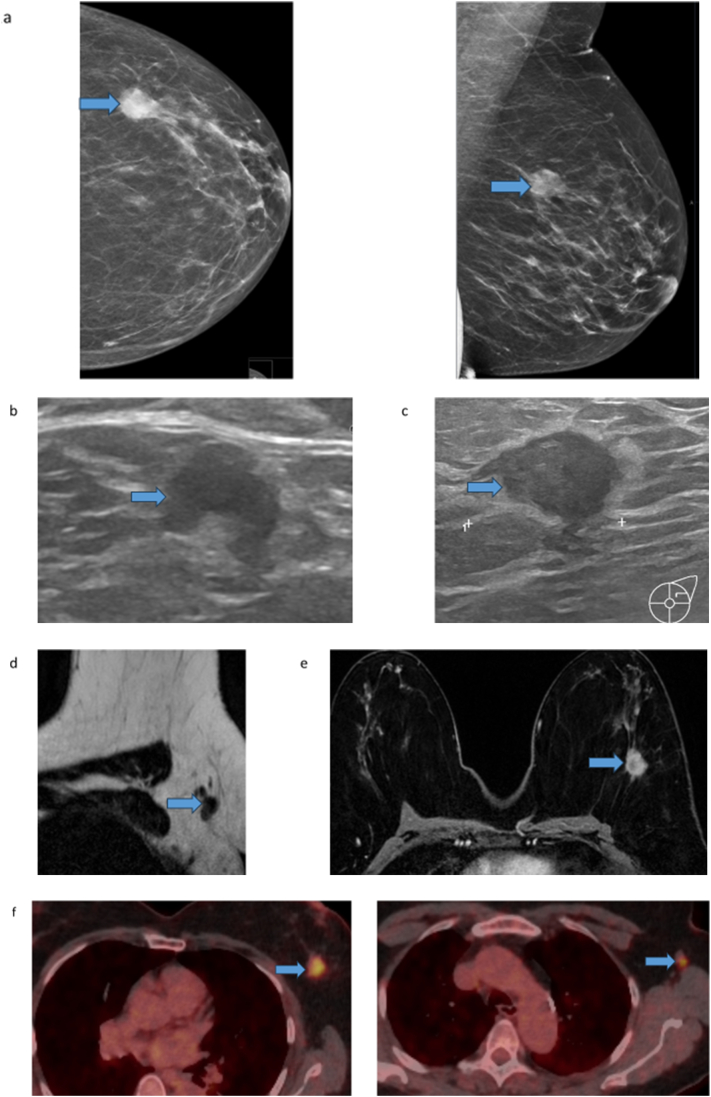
Mammography of the left breast shows a spiculated mass in the upper lateral quadrant in front and oblique view, suspicious of malignancy (blue arrow) (a). Ultrasound of the left axilla demonstrates a pathological axillary lymph node with a cortical thickness of 5 mm (blue arrow), which is highly suspicious for metastasis (b). Ultrasound of the left breast reveals a malignant irregular hypoechoic mass in the left breast (blue arrow) (c). MRI of the left axilla demonstrates a pathological lymph node with cortical thickness (blue arrow) (d). MRI of the left breast shows a spiculated malignant contrast-enhanced mass in the left breast (blue arrow) (e). PET/CT shows high FDG uptake in the tumor of the left breast and a lymph node in the ipsilateral axilla (blue arrow) (f).
